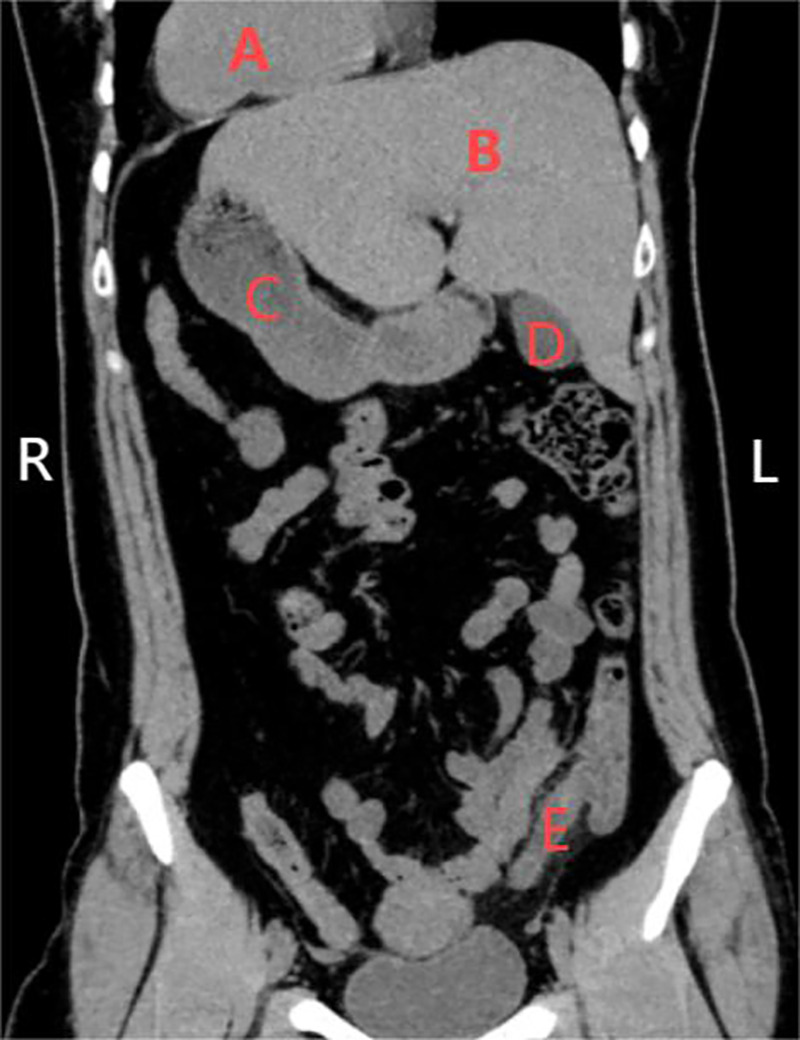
CT scan results upon admission. In the figure: (A) is the heart, (B) is the liver, (C) is the stomach, (D) is the gallbladder, and (E) is the appendix, which is inflamed.
